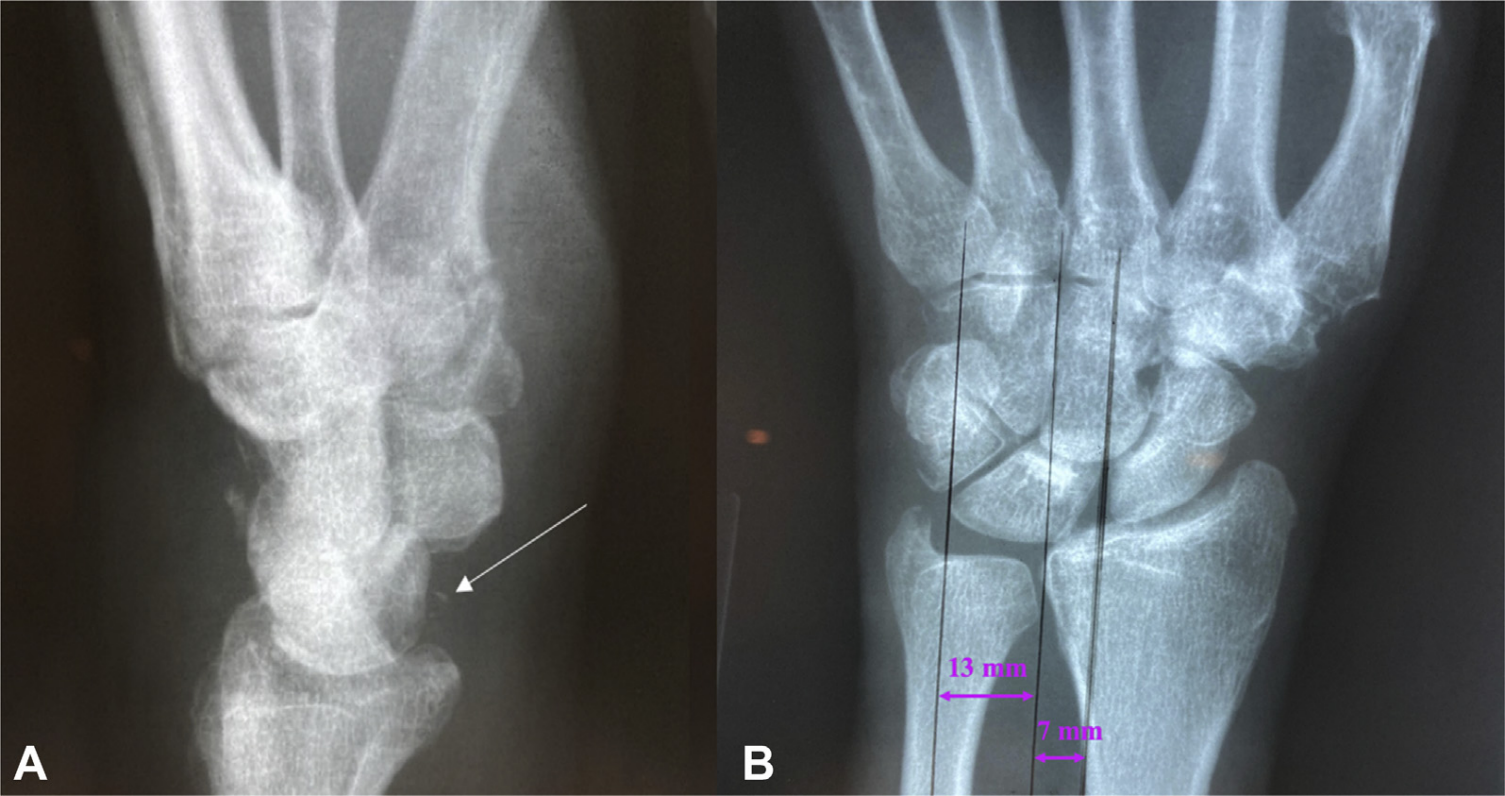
Radiographs of the left wrist taken at 7 weeks after the initial injury. A Lateral view. Volar fleck is identified by the white arrow. B PA view. Lunate uncovering 65%.
